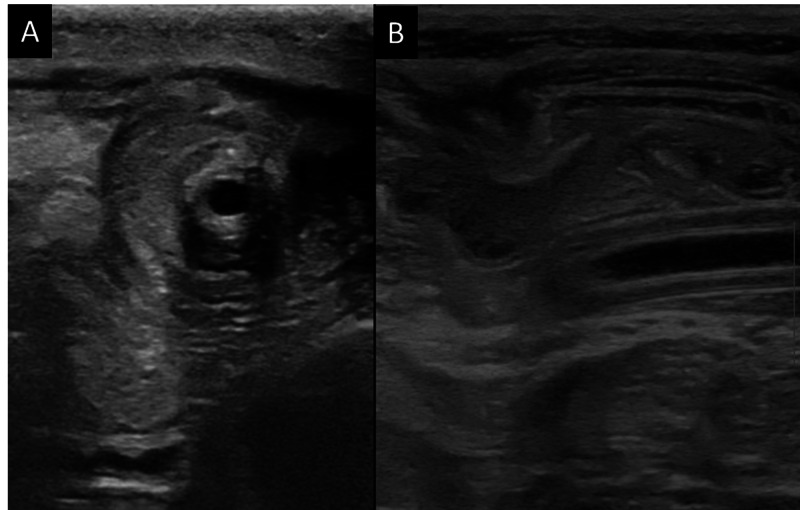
(A) Ultrasound transverse images showing “target” or “bowel within bowel” or “doughnut” appearance of bowel classical for intussusception. (B) Longitudinal scan shows “sandwich” appearance with tube as the leading point.
